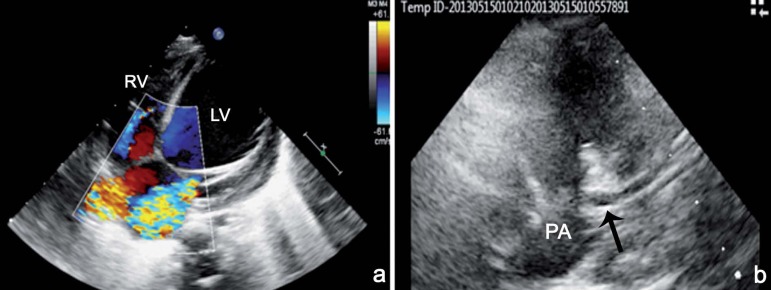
A - Apical four chamber view echocardiogram showing dilated left
